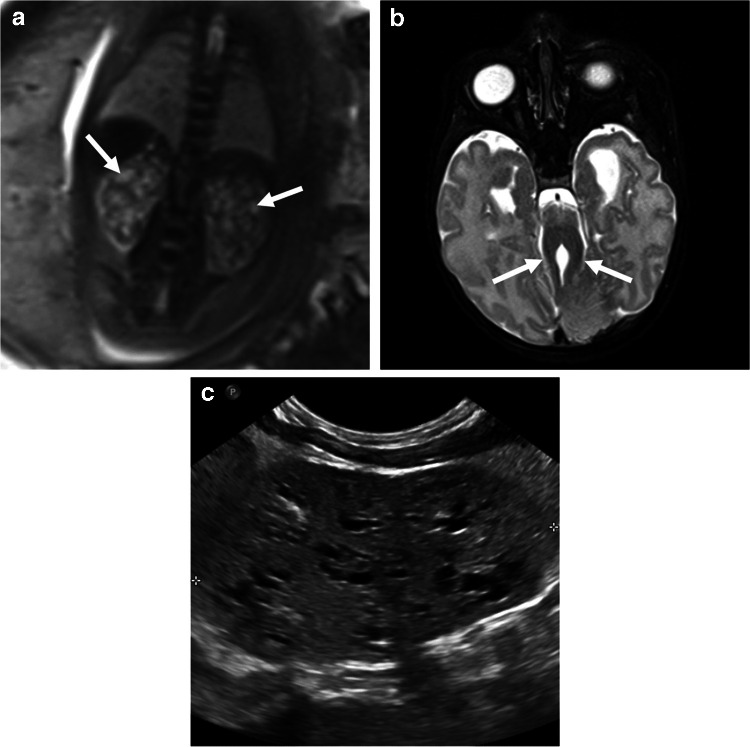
a Coronal Single-Shot Fast Spin Echo (SSFSE) MRI of a 31-week gestation female fetus with postnatally confirmed Joubert syndrome, a ciliopathy, demonstrating cystic renal dysplasia with bilateral innumerable T2- hyperintense renal cysts (arrows). b Axial T2 with fatsaturation MRI of the postnatal brain shows elongated superior cerebellar peduncles (arrows) resulting in the molar tooth appearance typical of Joubert syndrome. c Postnatal longitudinal ultrasound showing the dysplastic appearance of the left kidney. The right kidney (not shown) had a similar appearance
